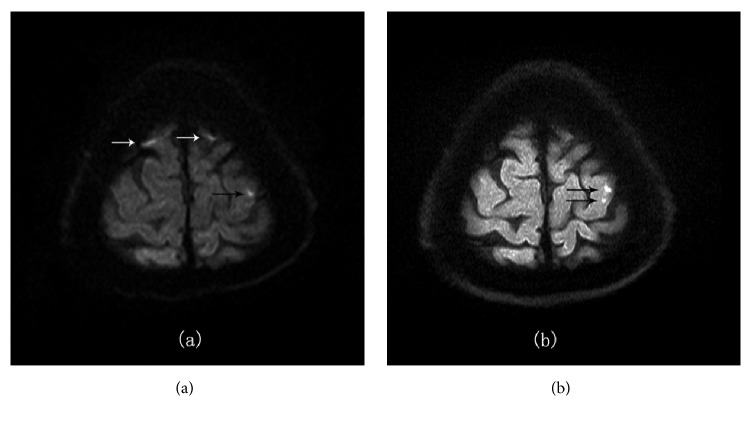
A 46-year-old male patient with acute cerebral infarction showed increased specificity and sensitivity of rs-DWI. (a) On ss-DWI image, there were 3 suspicious prominent subtle lesions. (b) On rs-DWI image, 2 lesions with white arrow did not appear, which should be artifacts from skull, whereas one lesion was confirmed to be true; even another small lesion was also found (black arrows).
